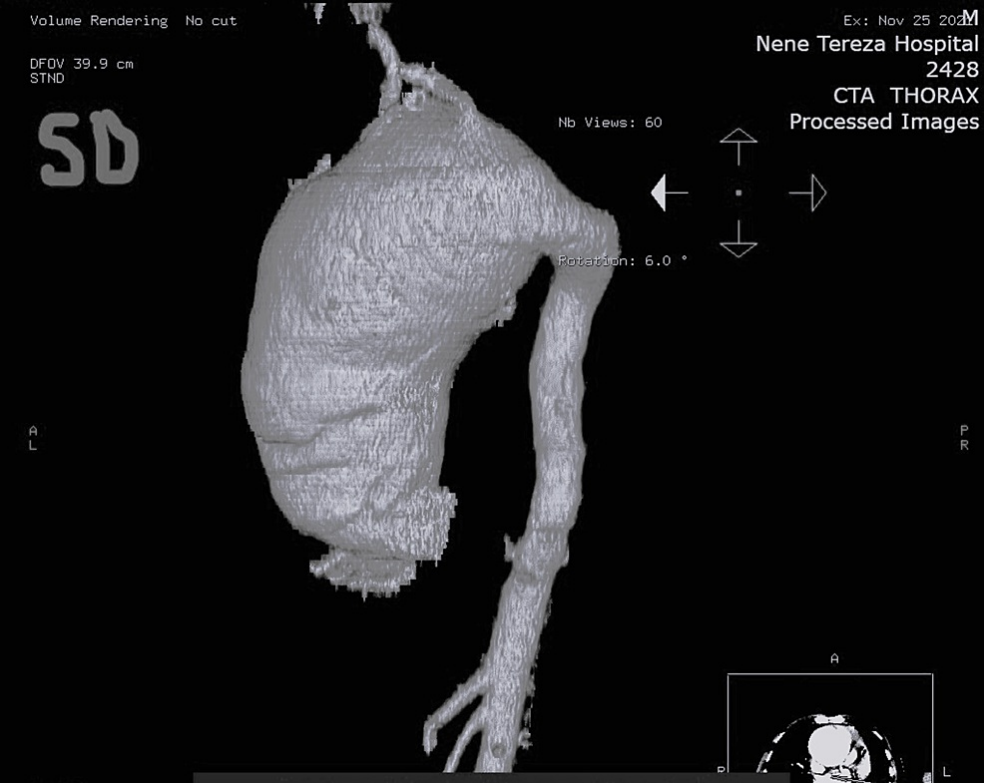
Computed tomography reconstruction of the thoracic aorta
